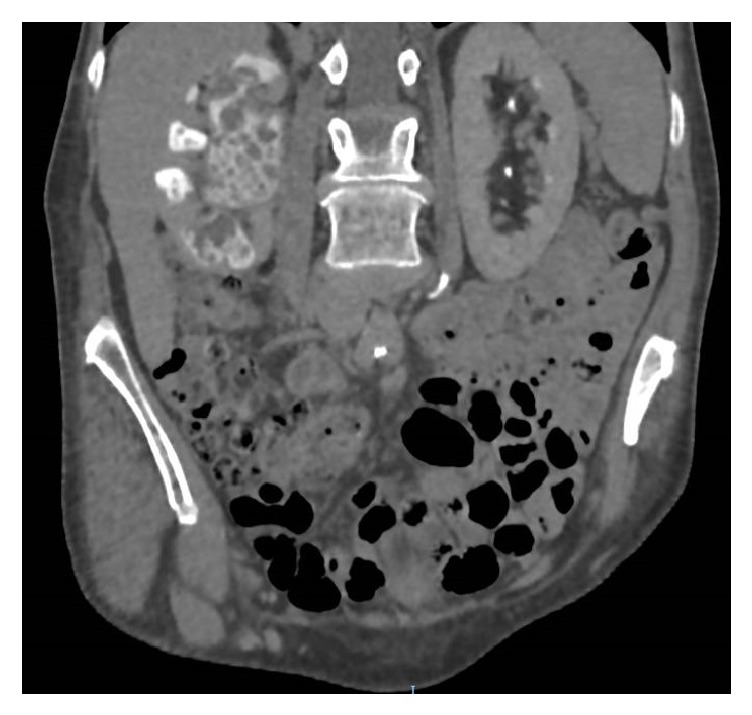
Contrast enhanced CT scan of renal tract, in coronal plane showing appearance of matrix stone throughout the right kidney.
